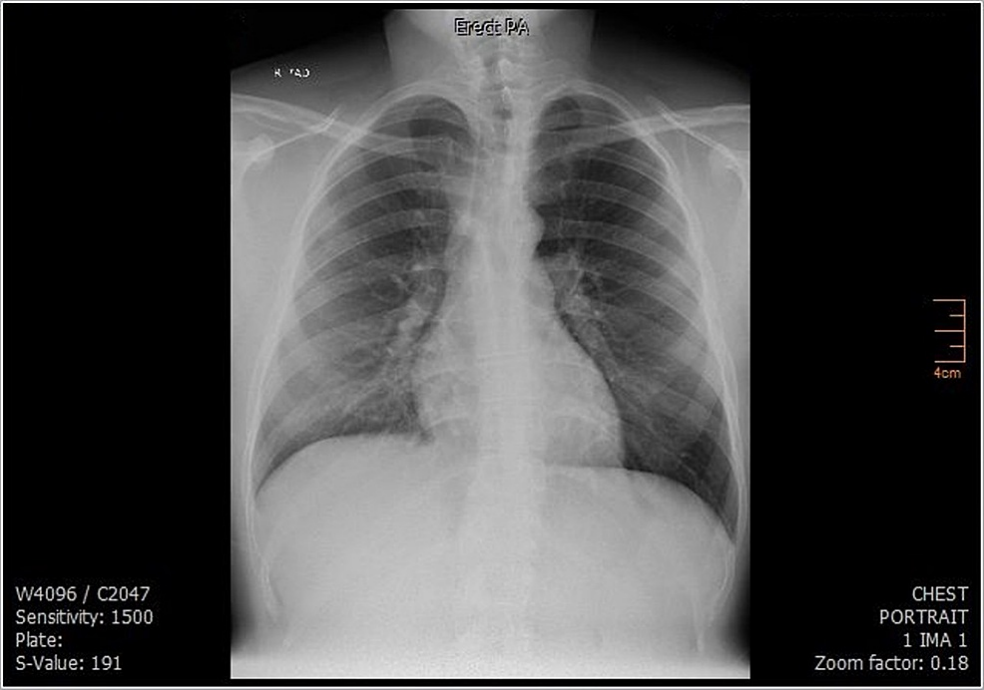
The first chest X-ray (CXR) of Mr. R. Chest X-ray done when Mr. R. presented to the emergency department, and it was normal.
